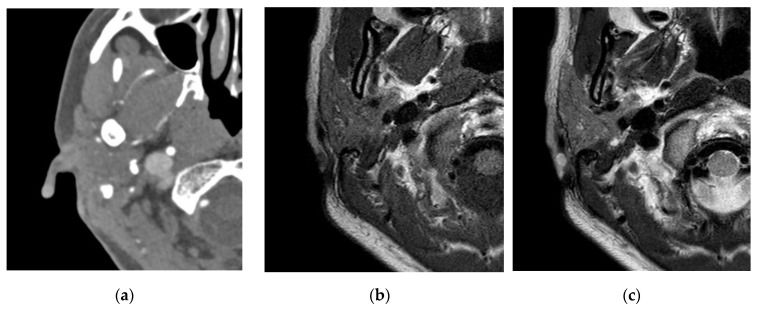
On brain computed angiography, an enhanced mass about 0.5 × 0.5 cm in size with soft tissue density was revealed at the helix of the right auricle. (a) Through neck magnetic resonance imaging (MRI), the mass at the helix of the right auricle was revealed with low signal intensity in T1-weighted images (b) and high signal intensity in T2-weighted images. (c) With suspicion of vascular tumor, surgical excision was planned upon patient request.
